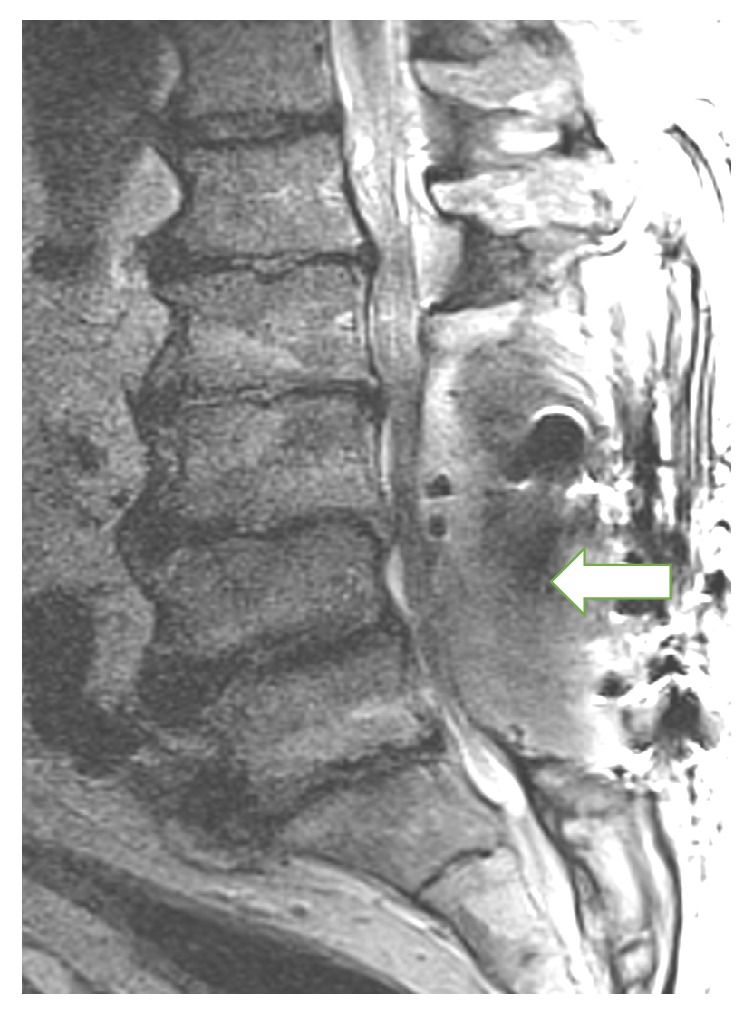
Lumbar spine MRI from July 6; arrow designates 12 × 3 × 3 cm abscess posteriorly with severe cord compression of the thecal sac, most prominent at the L4-L5 level (T2-weighted image provided by Craig Sherman).
